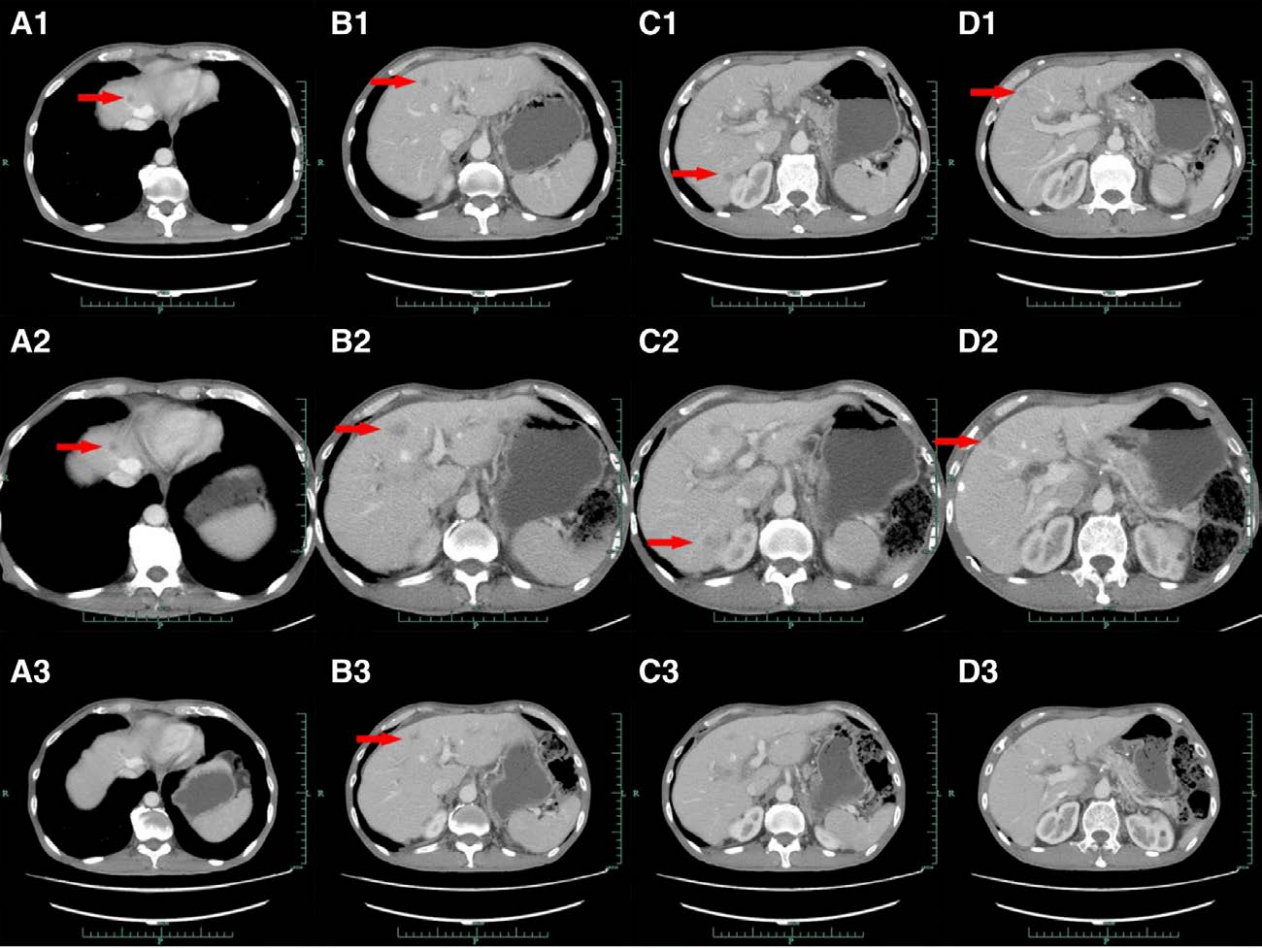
Abdomen CT scanning showed the metastatic tumors in the liver (red arrows). (A1—D1) April 24, 2020. (A2–D2) July 3rd, 2020. (A3–D3) August 17, 2020. CT = computed tomography.
